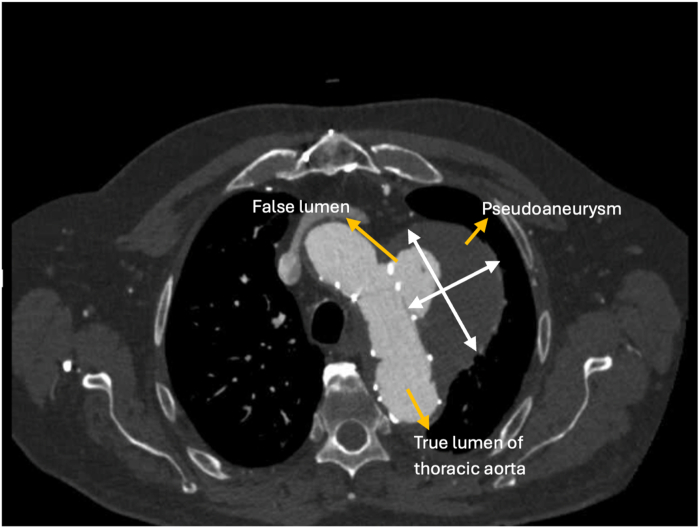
Computed Tomography With Contrast of the Chest Showing an Aortic Aneurysm in the Descending Aorta With a Thoracic Endovascular Aortic Repair Stent
